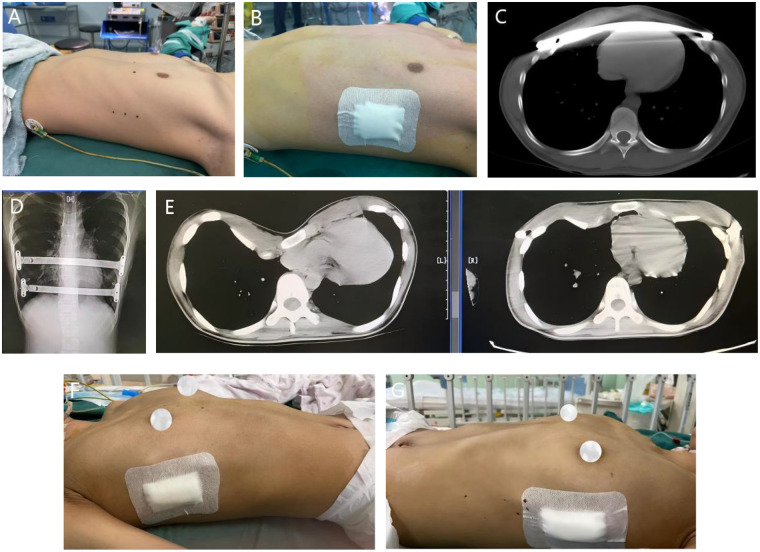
(A,B) show preoperative and postoperative chest photographs of a male patient. (C) shows postoperative chest CT imaging. (D,E) show intraoperative placement of double bars in a female patient with severe pectus excavatum, along with preoperative and postoperative radiographic images. (F,G) show postoperative chest photographs.
